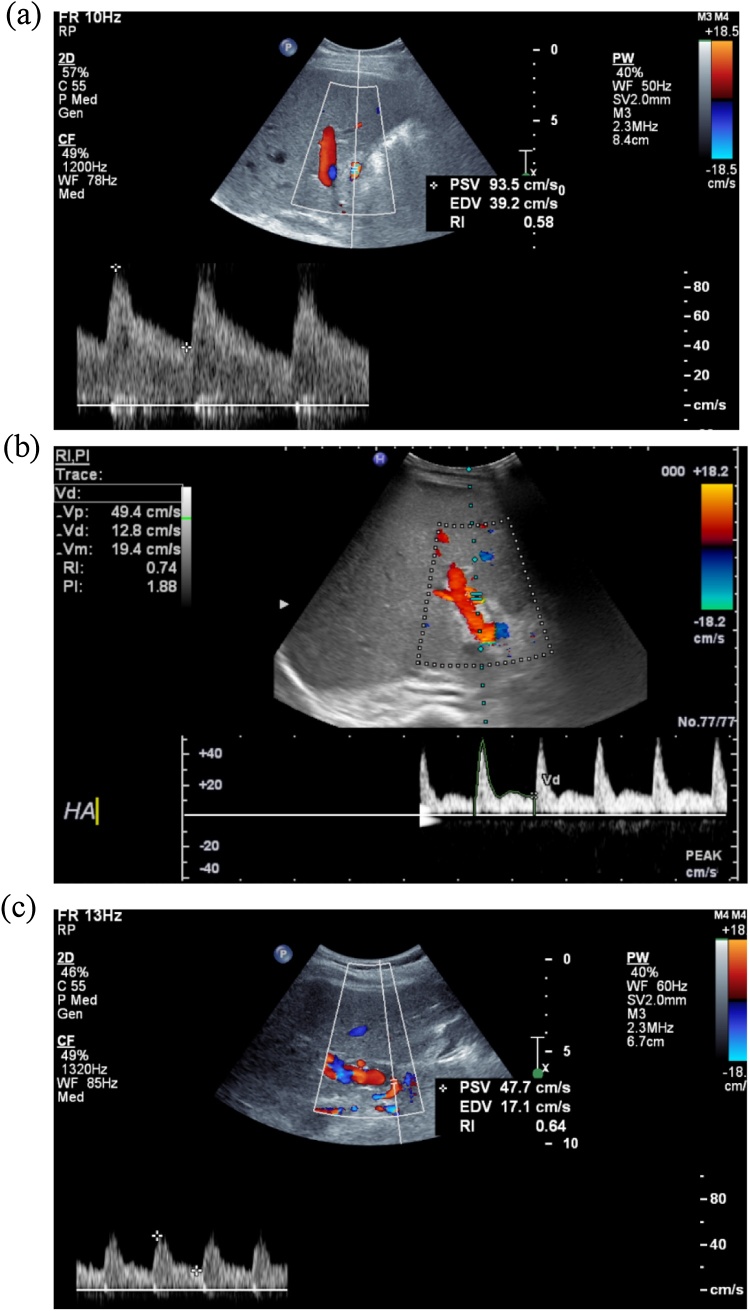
Colour-doppler-ultrasonographic images at late post-operative course; for case 1 (*panel a*) at post-operative day (POD) 38, case 2 (*panel b*) at POD55 and case 3 (*panel c*) at POD90. All scans showed adequate flows on the hepatic artery with resistance index within normal range.
